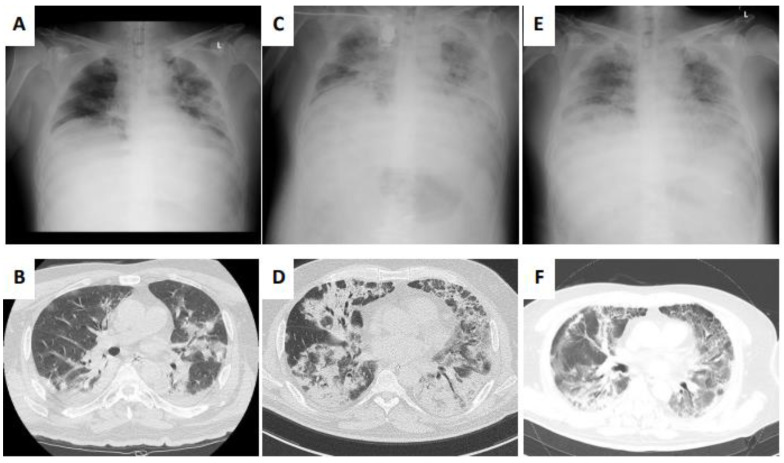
Chest images during the clinical course. (A) Chest X-ray and (B) computed tomography (CT) on admission. (C) Chest X-ray and (D) computed tomography (CT) at the time of SOP diagnosis. (E) Chest X-ray and (F) computed tomography (CT) on discharge.
